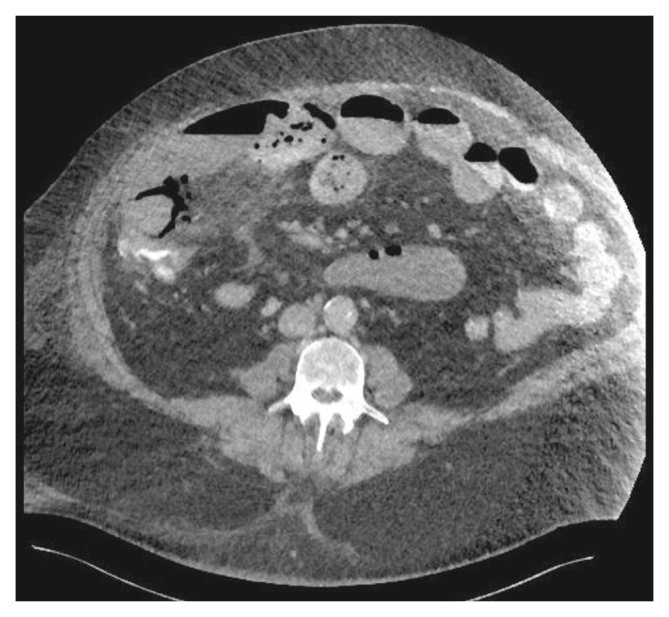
Abdominal computed tomography angiography of the patient revealed a distended bowel with pneumatosis intestinalis. Focally, the small intestine lacked coloration and showed infiltration of mesenteric fat tissue.
